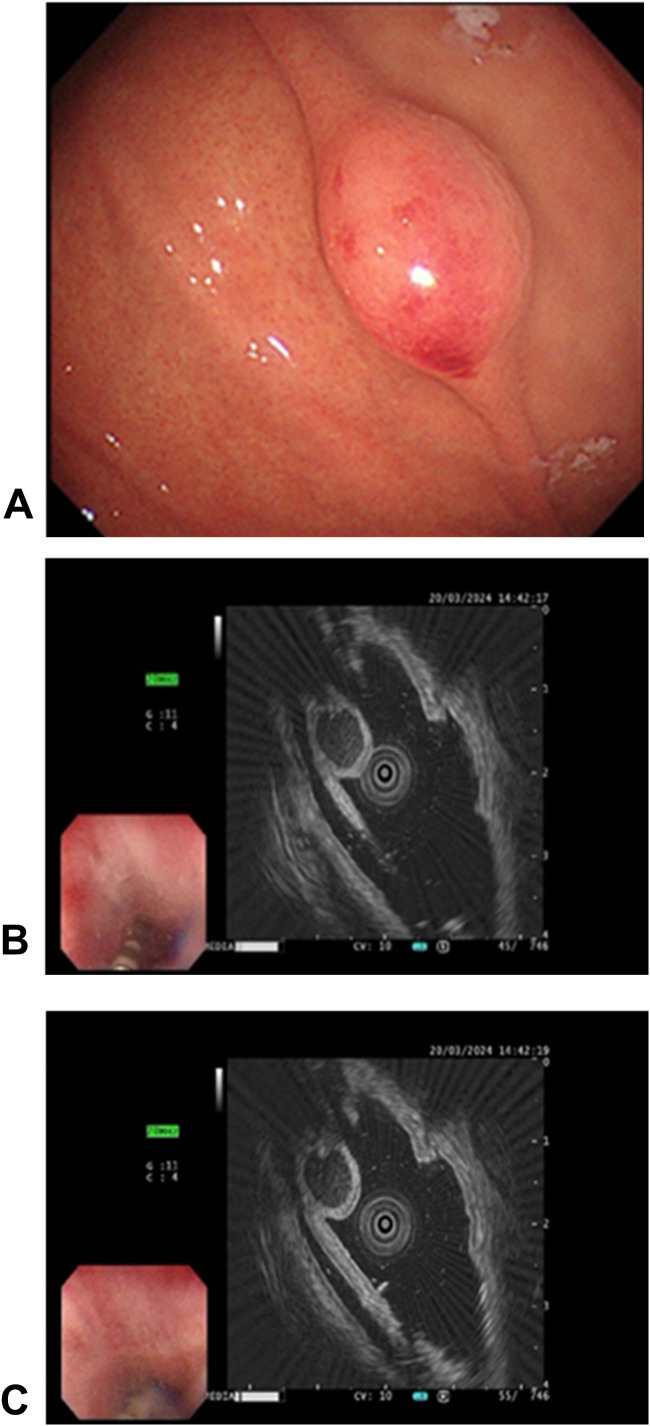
Endoscopic manifestations of the mass. (A) The gastric body exhibited a spherical, nontender submucosal elevation classified as type 0-Is according to the Paris classification of superficial gastrointestinal tumor lesions (0-Is: flattened, elevated type). The surface appears congested and reddish, indicating mucosal changes; (B, C) Endoscopic ultrasonography of the lesion. A mixed hypoechoic mass, characterized by inhomogeneous internal echogenicity, visible tubular structure, and posterior attenuation, is observed. The hemispherical mass extends into the cavity and originates from the submucosa.
